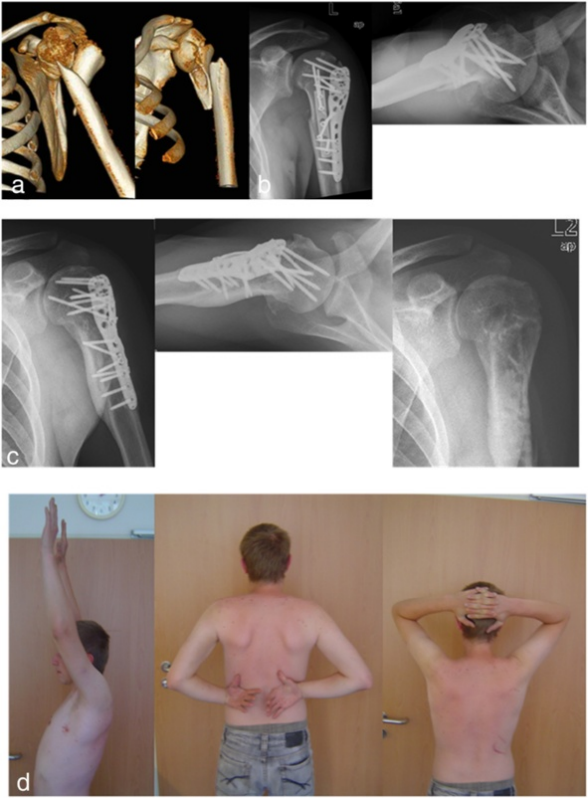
a 24 year old male with dislocated proximal fracture with metaphyseal comminution and intermuscular fragment. b postoperative x-ray showing anatomical reduction after double plate osteosynthesis. c X-ray after one year showing complete healing of the fracture, x-ray after material removal. d functional result after 2 years, Constant 90 points
